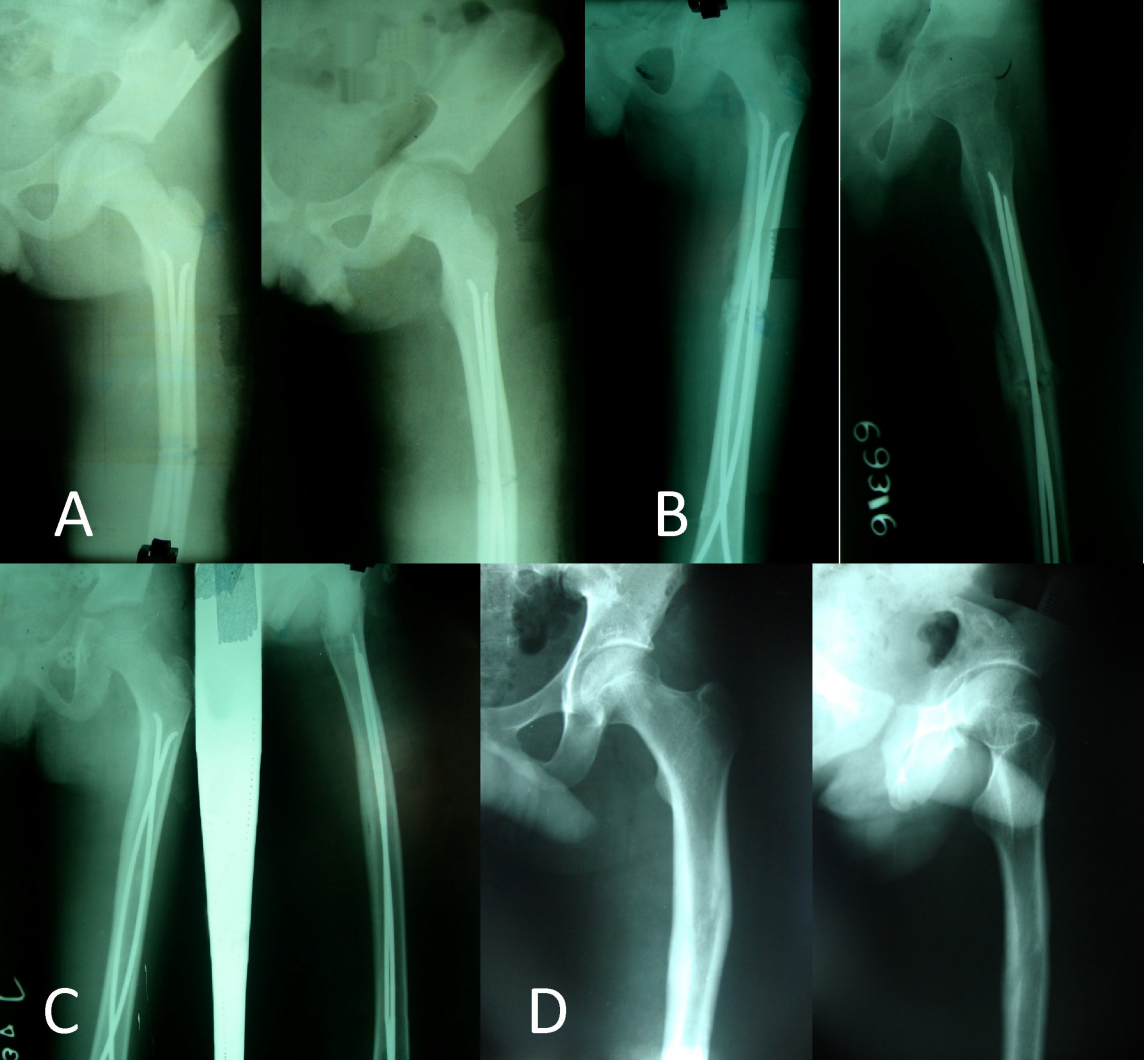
Radiographs of a 9 year old child with left sided femoral shaft fracture managed by titanium flexible nailing. (A) shows significant malalignment in both coronal (15° varus) and saggital plane (10° anterior apex). Fracture alignment improved slightly during follow up (B, C) and at 7 years significant malalignment still remained in coronal plane (15°) but not in saggital plane.
